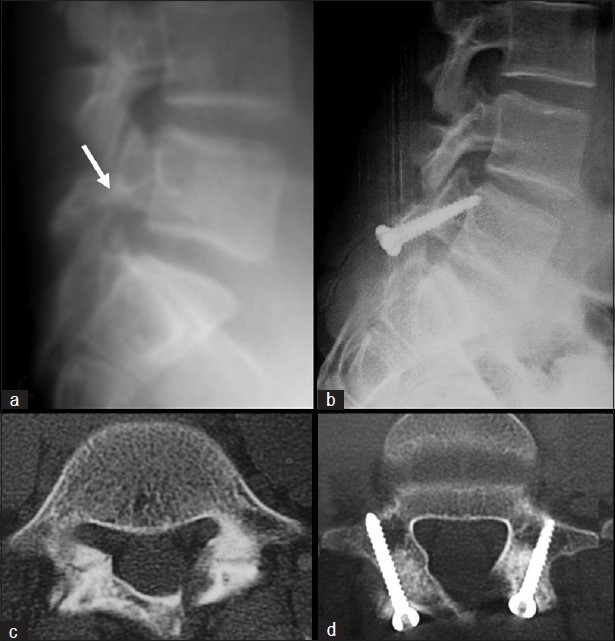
(a) Preoperative lateral radiograph and (b) follow-up lateral radiograph of a patient with bilateral spondylolysis of L5 fixed by Buck’s technique on both sides. (c) Preoperative and (d) follow-up axial CT scan of the same patient showing complete healing of the pars defect bilaterally, without signs of screw loosening, back out, or breakage
